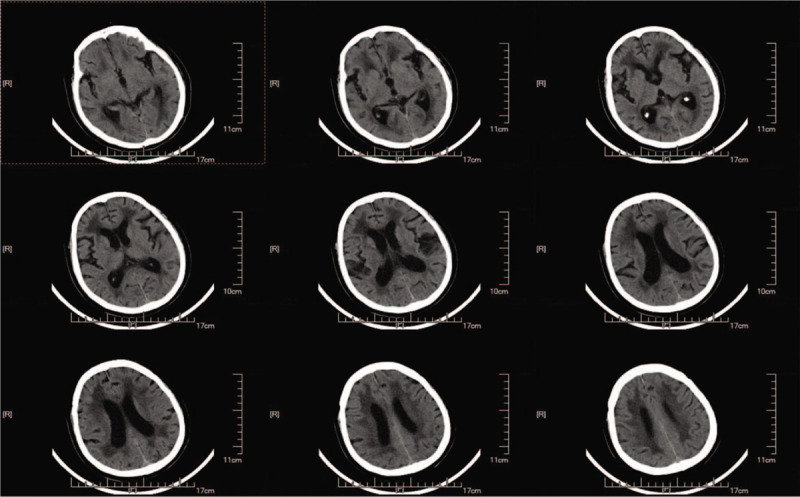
Preoperative head computed tomography scan obtained during first admission indicated ventricular enlargement and obvious paraventricular edema.
